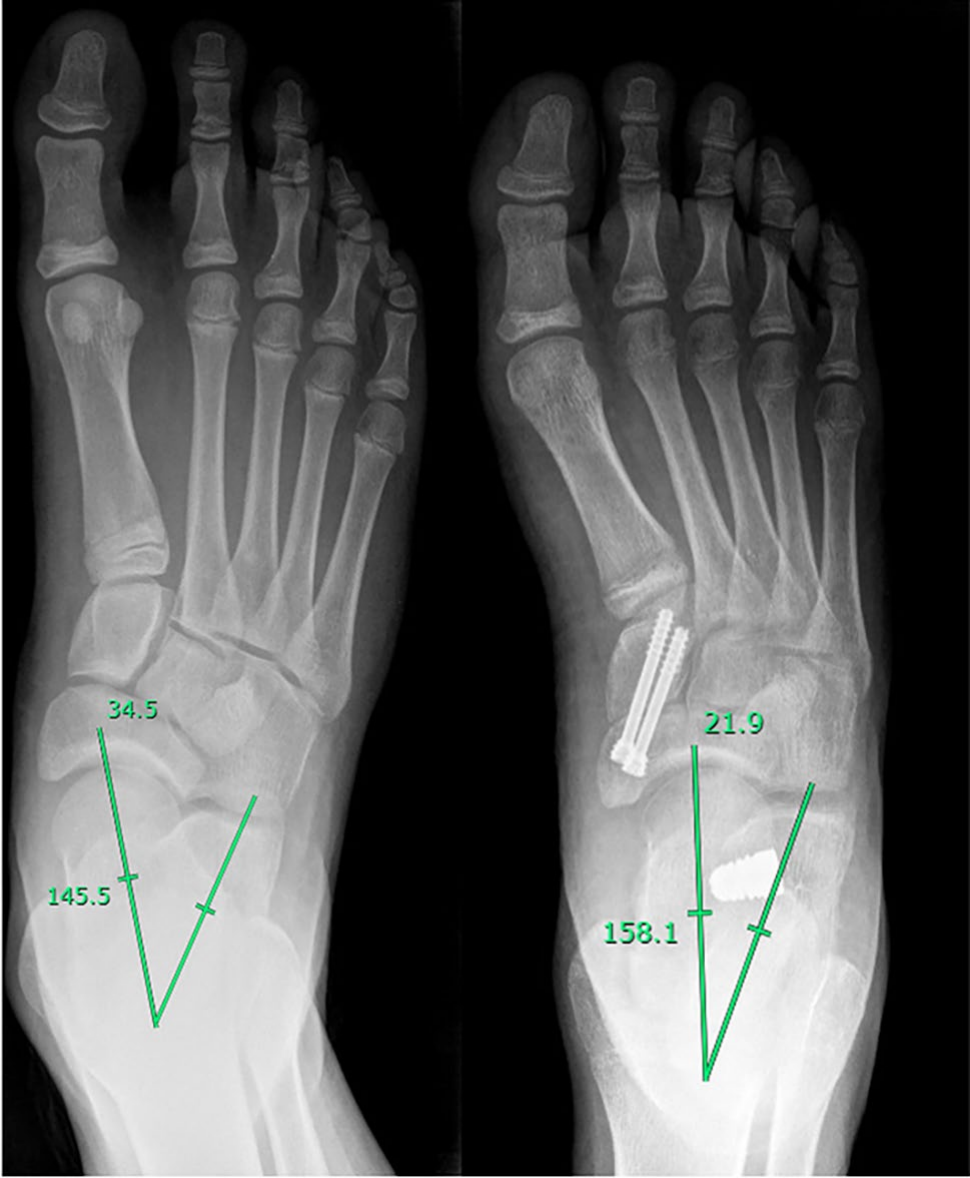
Evaluation of kite angle in a pre (left panel) and post (right panel) surgical x-ray in a left foot
